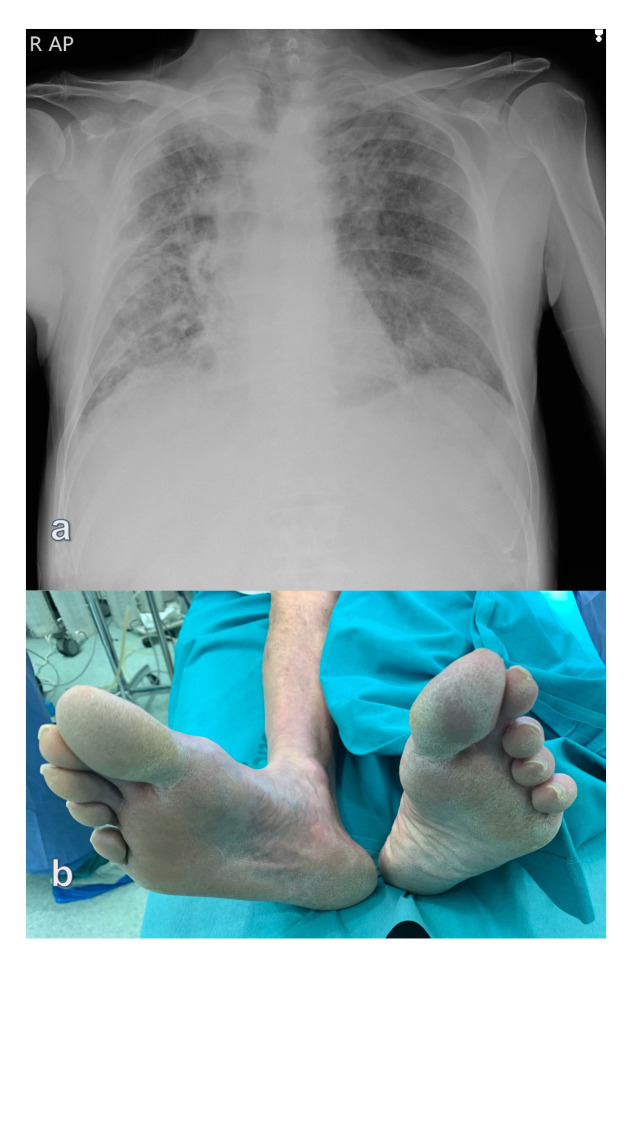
(a) Chest X-ray revealing bilateral peripheral ground-glass lung opacities (typical feature of COVID-19 pneumonia) as well as multifocal air-space disease; (b) Preoperative picture showing patient’s ischemic foot which required immediate revascularization (patient #2).
